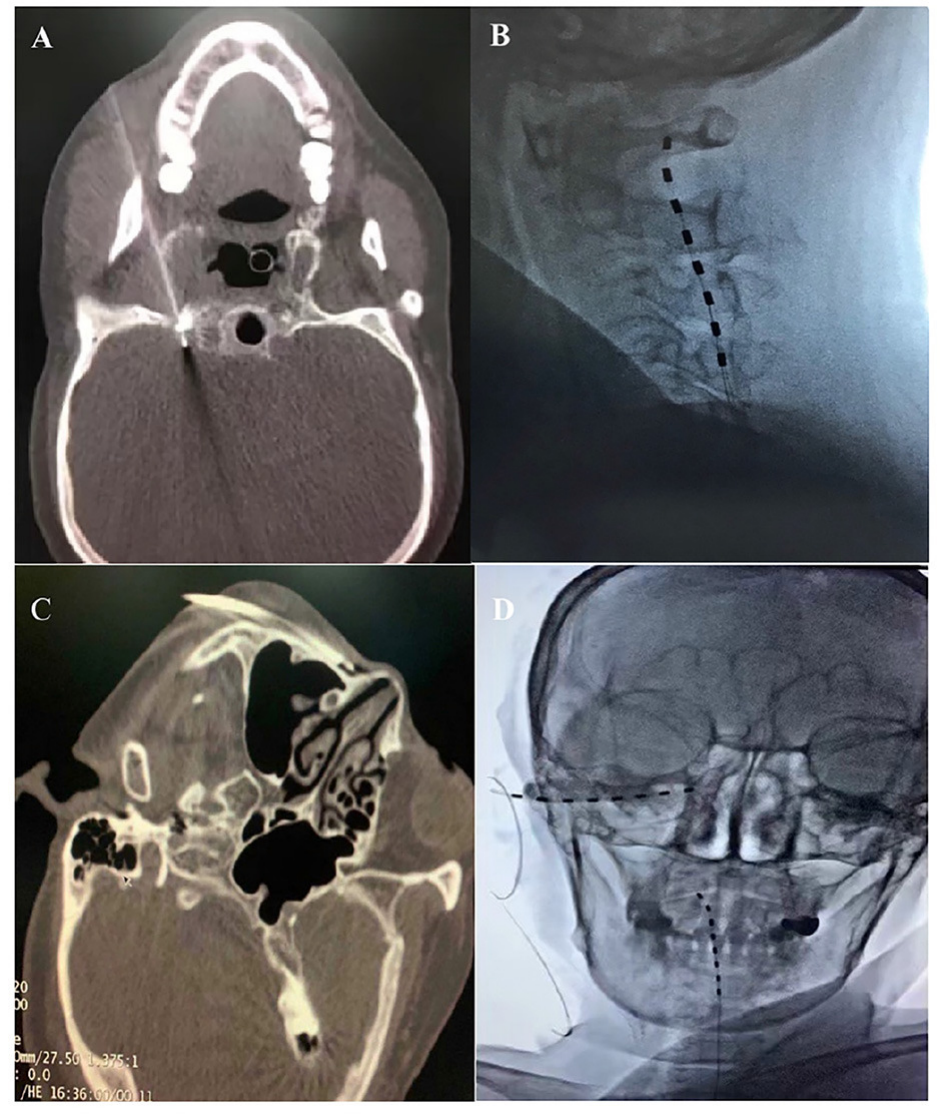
(A) CT-guided PRF on the Gasserian ganglion. (B) High cervical SCS at the C1-C2 level. (C) A 14-G needle inserted at the level of the right zygomatic bone. (D) High cervical SCS combined with infraorbital nerve (ION) PNS.
